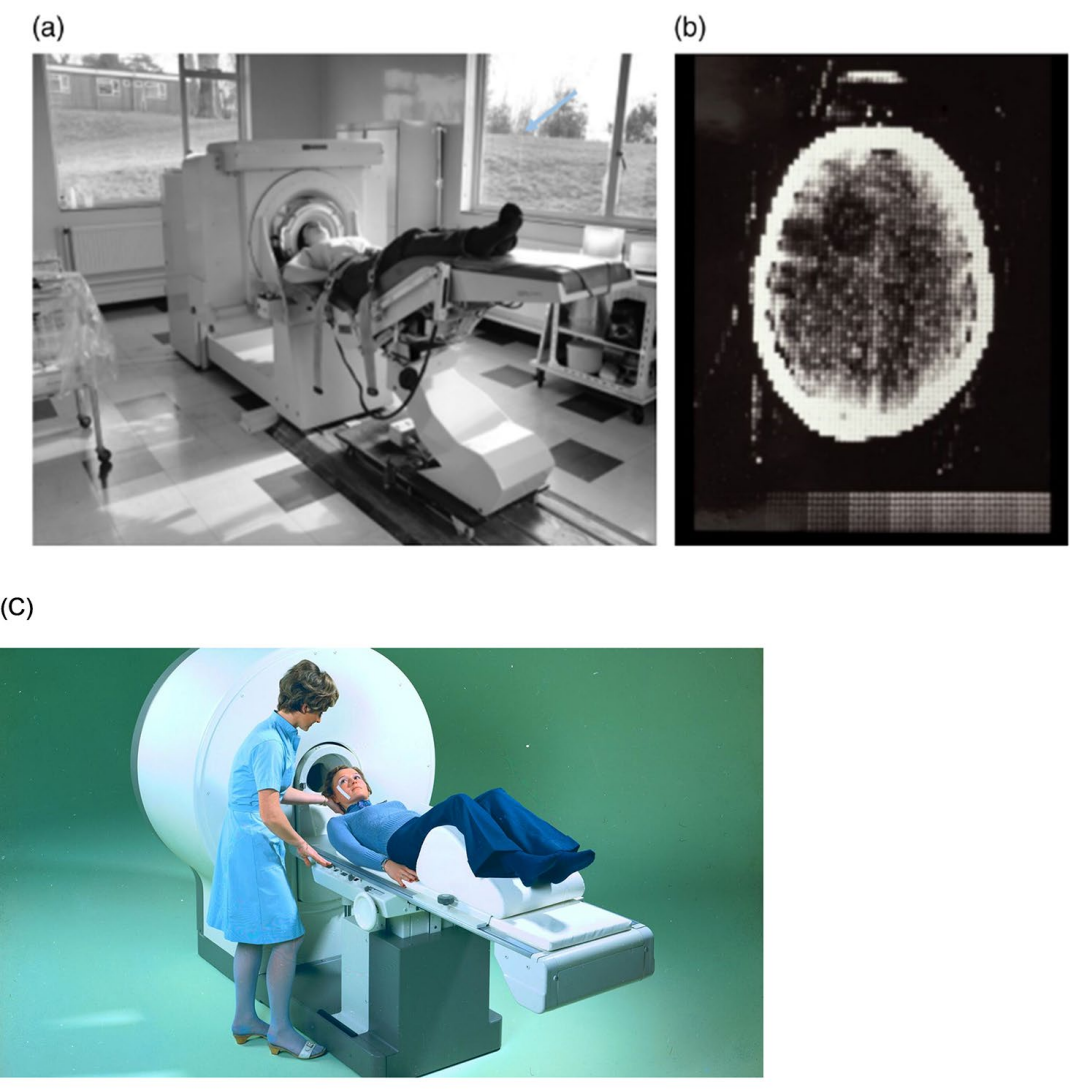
First EMI Scanner (a), first CT scan of the brain (b), and Siemens prototype CT Scanner Siretom (c), Siemens Healthcare GmbH, Erlangen, Germany
